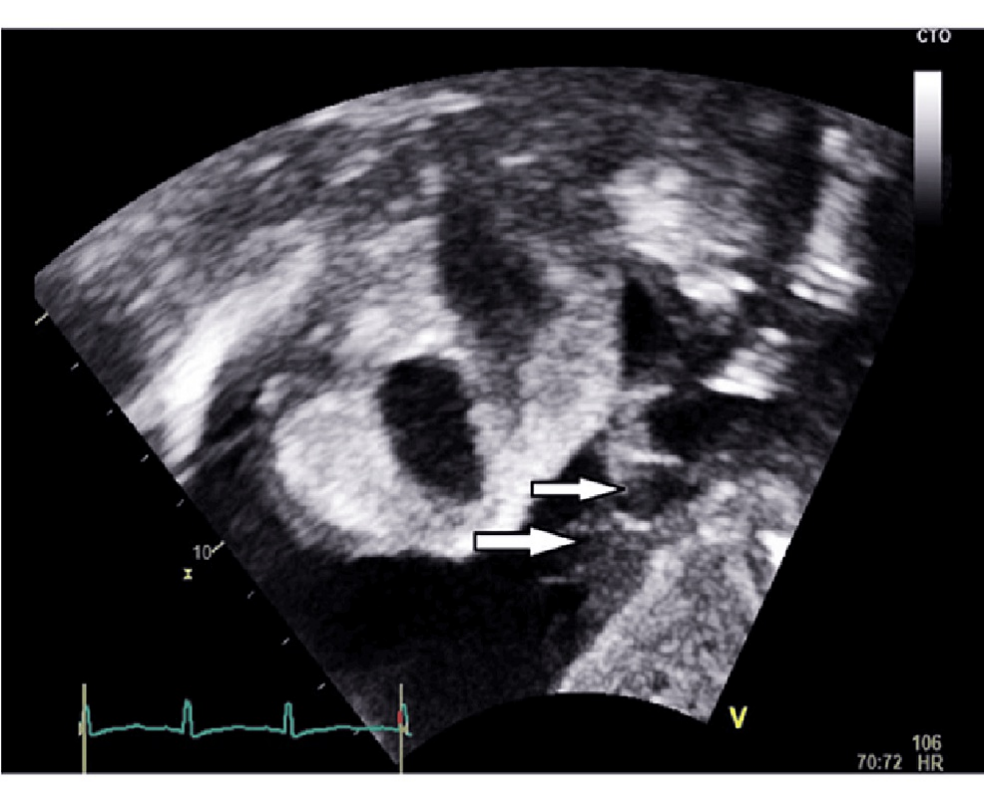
Echocardiogram showing the fibrin strands in the pericardial effusion (arrows) that represent the inflammatory process
